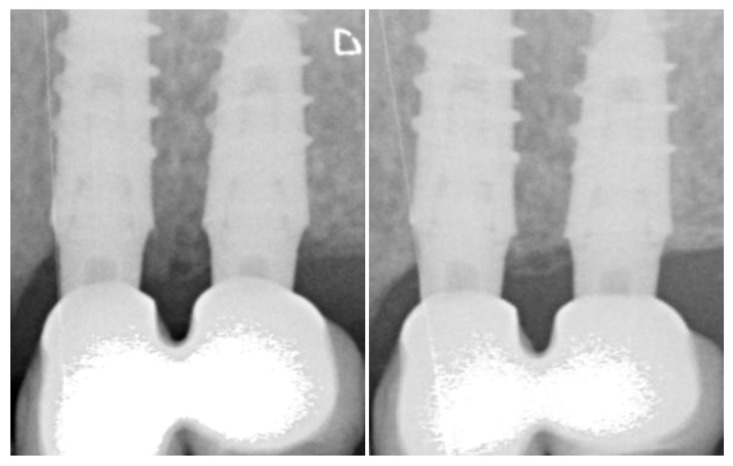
Radiographic appearance of the marginal bone levels at adjacent implants loaded with tapered abutment at loading (left) and one year after (right).
